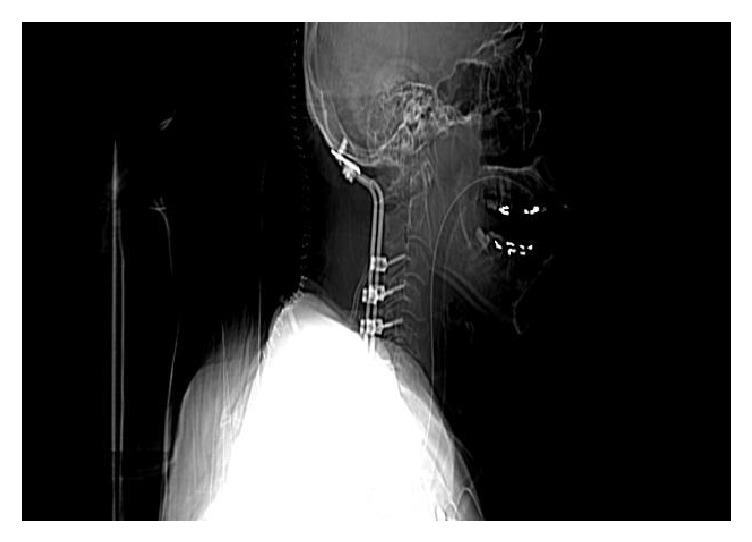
CT cervical spine after surgery showing removal of the tumor.
